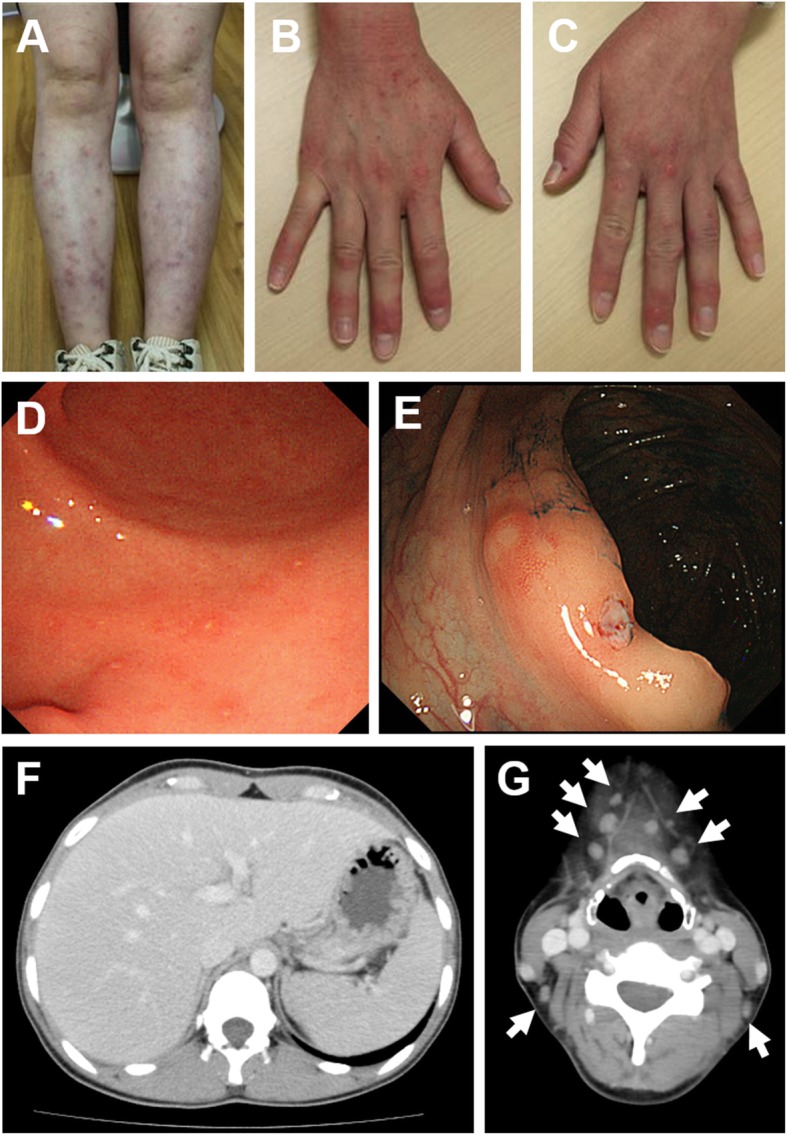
Skin and gastrointestinal lesions of patient 2. a Erythema nodosum in the bilateral lower extremities. b, c Pernio-like rash in the fingers. d, e Endoscopic findings at 29 years of age; d multiple ulcers in the gastric mucosa and e ulceration in the ileocecum. f, g CT findings at 29 and 33 years of age; f hepatosplenomegaly and g generalized lymphadenopathy. White arrows indicate swollen lymph nodes
